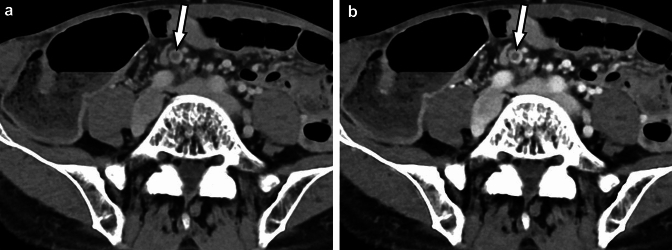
A small thrombus in the mesenteric vein (arrows) is visualized on the photon-counting CT images at 70 (A) and 50 keV (B). The 50 keV image (B) shows the thrombus with higher contrast than the 70 keV image (A). Photon-counting CT is excellent for visualization of venous thrombus
